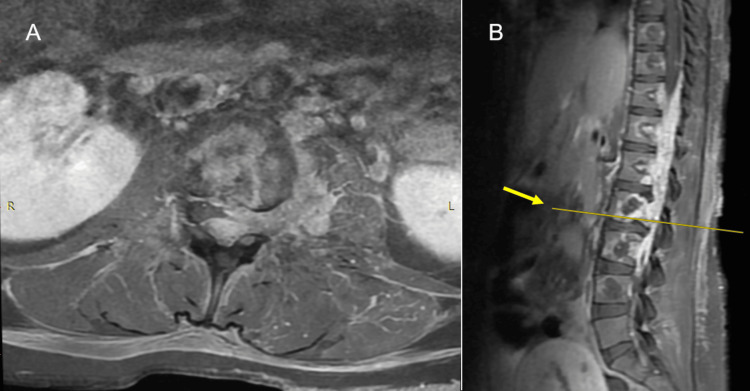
Axial and sagittal MRI at L2 level (A) Axial T1-weighted MRI using gadolinium-based contrast agent showing the involvement of the vertebral body, an epidural occupational lesion causing displacement to the right of the cauda equina, and extension to the left neuroforamen L2-L3 with the involvement of ipsilateral psoas muscle. (B) Sagittal T1-weighted MRI using gadolinium-based contrast agent showing the extension of the lesion into the craniocaudal axis from its lower portion from T10 to L3 (arrow and line indicate L2 level) MRI: magnetic resonance imaging
